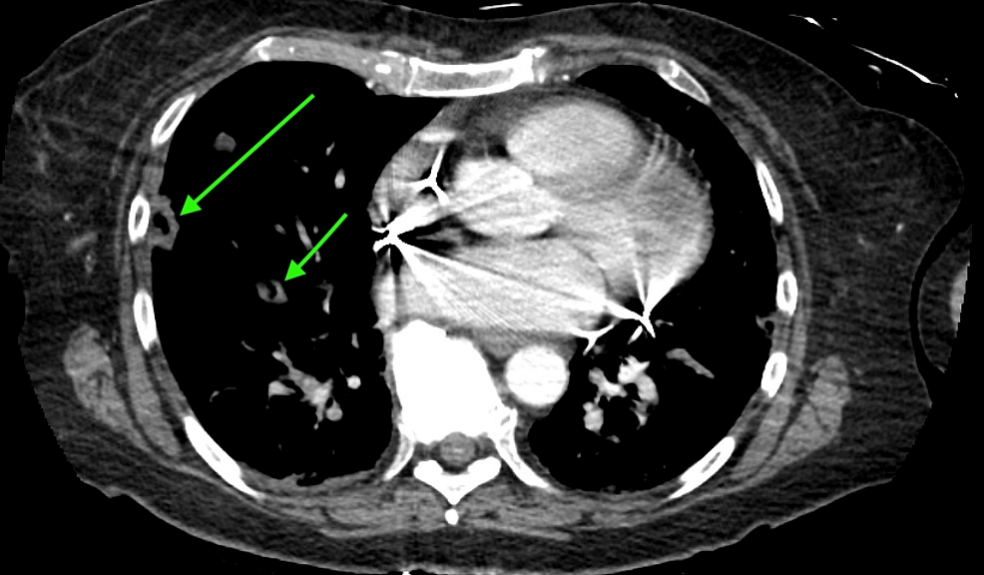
Lung CT, transverse cut, with cavitation suggestive of septic pulmonary emboli. CT: computed tomography
